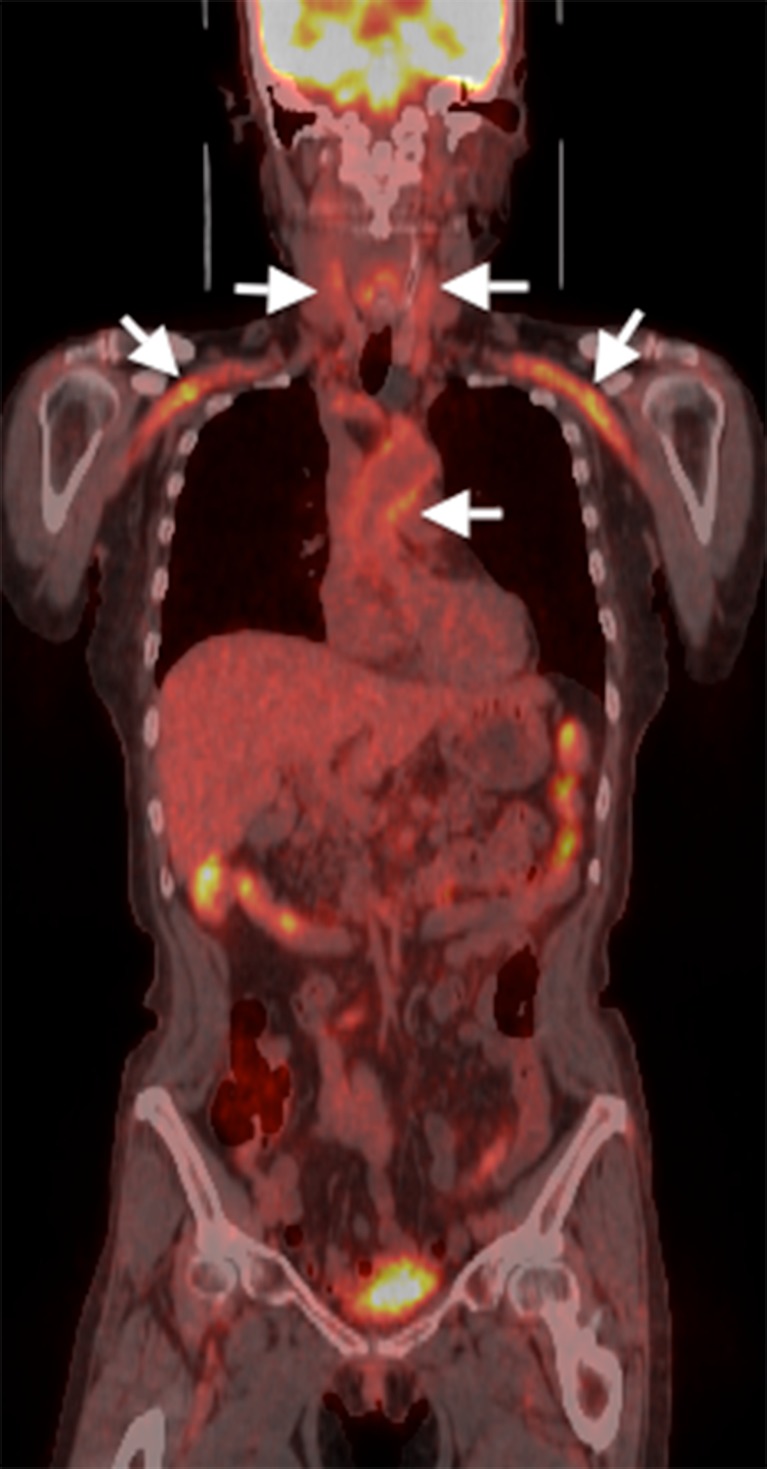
FDG-PET image with arrows pointing toward abnormal uptake in the carotid and subclavian arteries, as well as the thoracic aorta.
